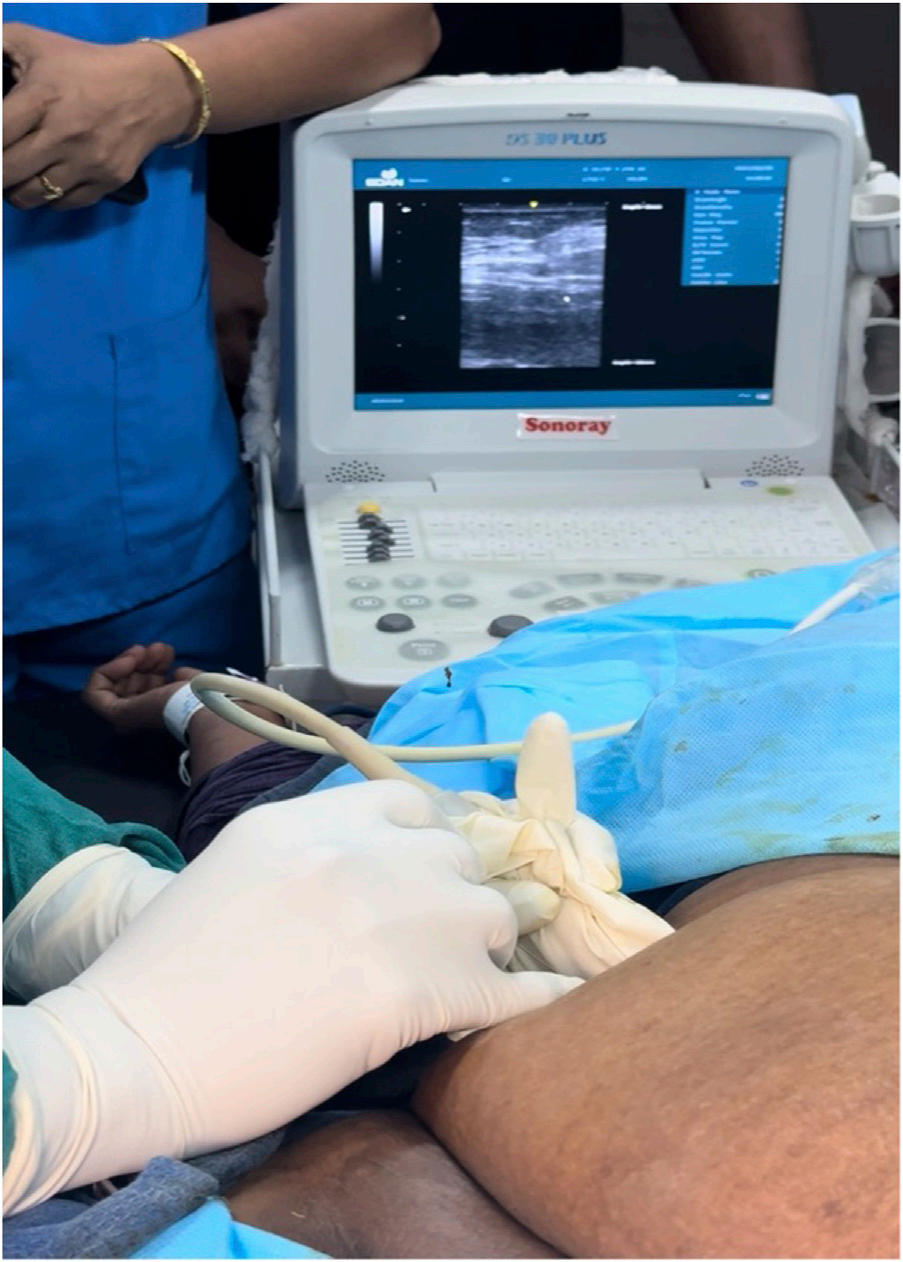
Intraoperative picture of injection of BT into the abdominal musculature, with Ultrasound showing three layers of abdominal muscles in the background.
